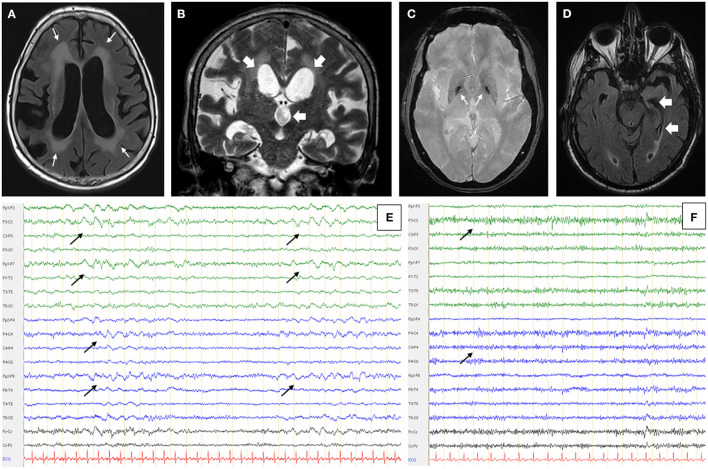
Patient's MRI and EEG. Patient N°1 brain MRI in axial FLAIR (fluid attenuated inversion recovering) sequence (A) and coronal T1 weighted sequence (B) showed periventricular confluent leukopathy (thin white arrows - A), cortical and subcortical atrophy predominant on the right hemisphere and a significant dilatation of lateral and third ventricles (large white arrows - B). (C) Patient N°2 had a hyposignal of the two pallidal tips in axial T2* sequence (white arrows). (D) Hippocampal atrophy, predominant on the left side, on patient's N°3 axial FLAIR MRI (white arrows). All MRI were performed on 3 Tesla devices (Magnetom Skyra, Siemens Healthcare, Erlangen, Germany and Achieva 3.0 T, Philips, Amsterdam, Netherlands). (E) Patient N°1 also had recurrent bilateral anterior delta waves on a 6.5 Hz basic rhythm (black arrows). (F) Patient N°2 had a non-pharmacological, bilateral and anterior fast rhythm at 14 Hz (black arrows). EEGs were performed and read on Deltamed devices and software (Natus Neurology, Pleasanton, USA).
